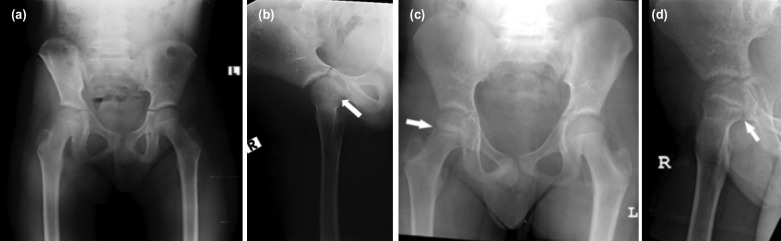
(a, b) Plain radiographs of the pelvis done immediately after the fall. The fracture was not obvious on the anterior-posterior radiograph but was visible on the lateral radiograph (arrow). (c, d) Plain radiographs five months after injury showing the posterolateral tension-type fracture in the right femoral neck (arrow).
